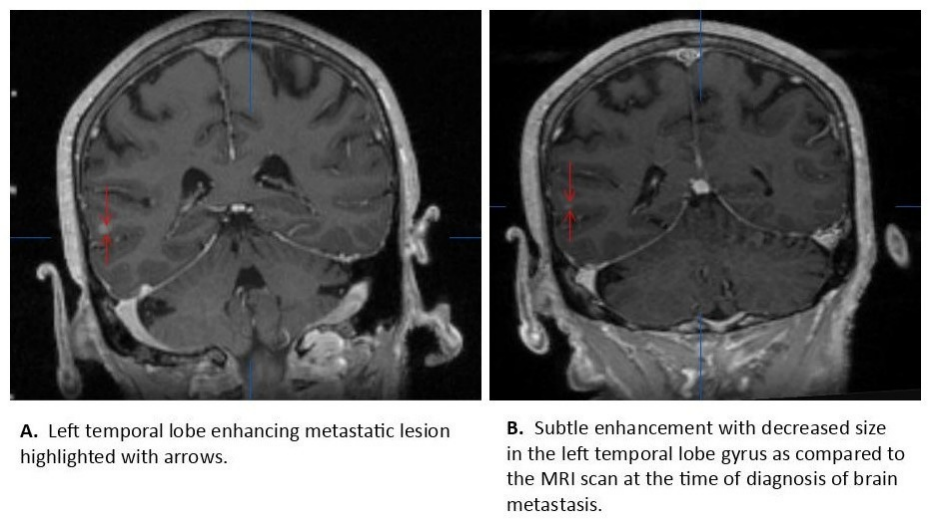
MRI scan of the brain from the brain lab, (A) at the time of the diagnosis or before SRS and (B) after SRS. MRI: Magnetic resonance imaging; SRS: Stereotactic radiosurgery.
